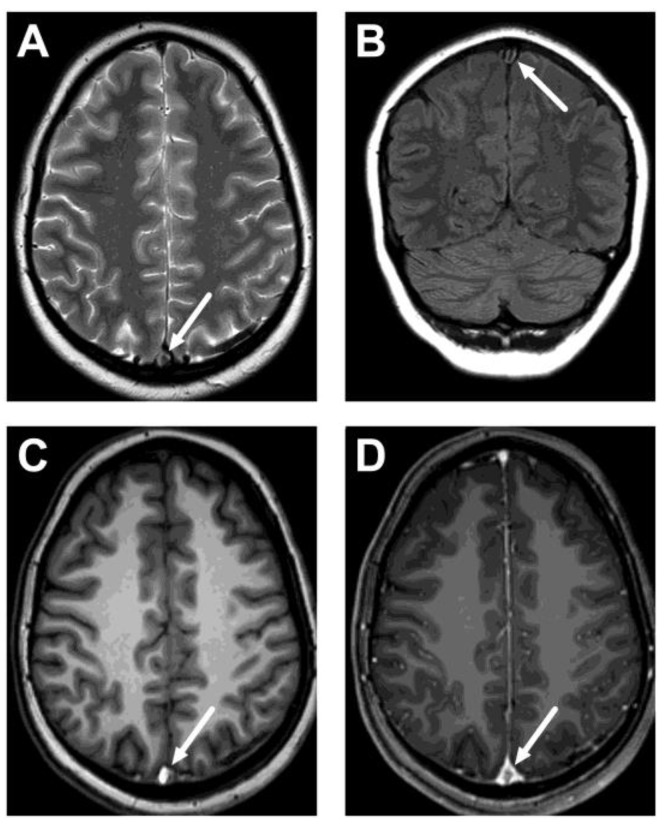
An example of cerebral venous sinus thrombosis on routine pre-contrast images. Images show a lack of normal flow void on T2-weighted (A) and fluid-attenuated inversion recovery (FLAIR) (B) images, as well as high signal on the pre-contrast T1-weighted image (C), in the superior sagittal sinus (arrows). Post-contrast T1-weighted image is provided for reference (D), showing the non-enhancing thrombus (arrow). Most patients did not show thrombosis on all routine sequences, however. The patient is the same as in Figure 1C.
